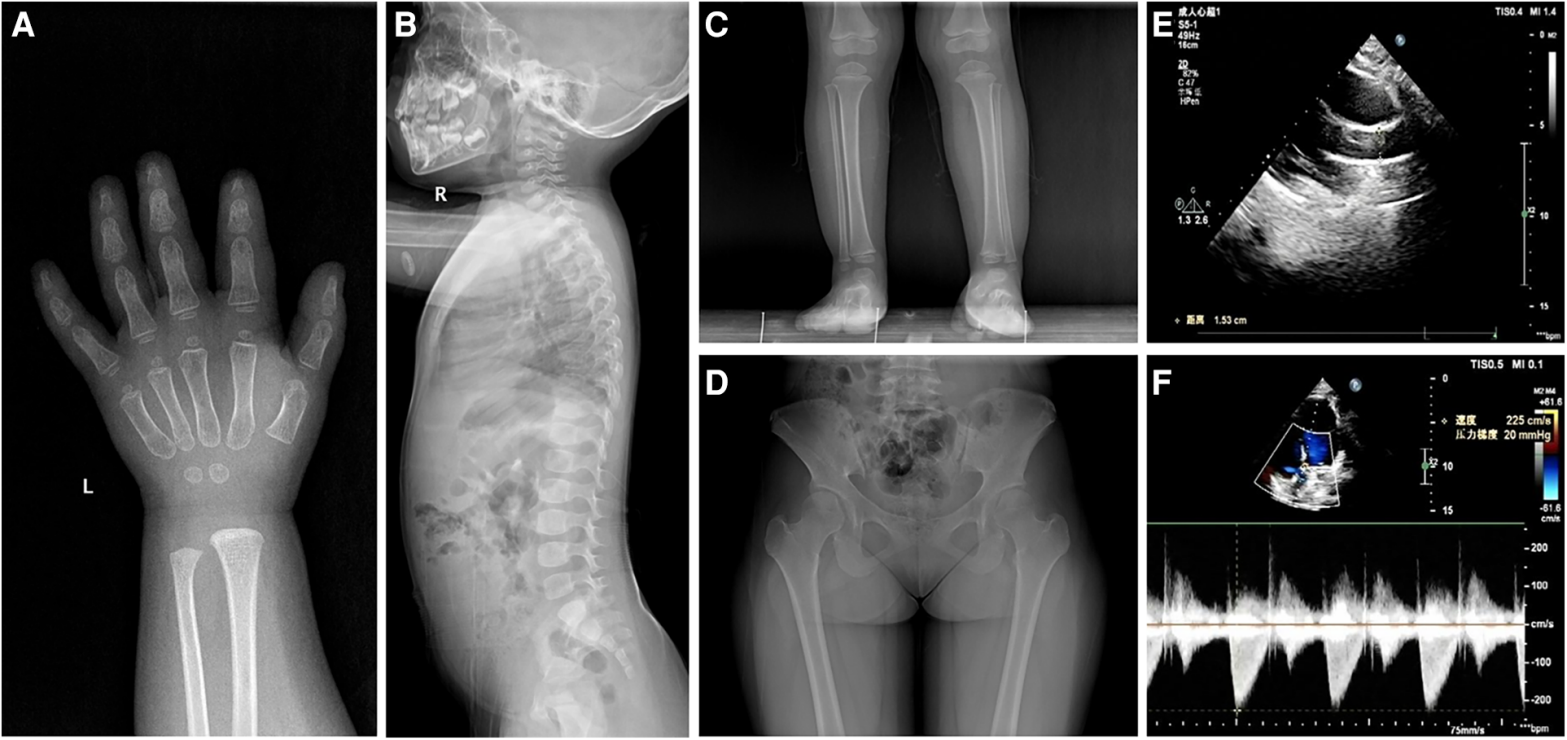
Radiological images showing (A) the internal notch in the second metacarpal and the external notch in the fifth metacarpal, (B) ovoid vertebral bodies, (C) cone-shaped epiphysis of the left leg in patient 1, and (D) hip dysplasia and the internal notch of the femoral heads in patient 2. Ultrasound images showing (E) aortic valve stenosis with an inner diameter of 15 mm and (F) a mild increase in aortic outflow of 2.2 ms^−1^ in patient 2.
